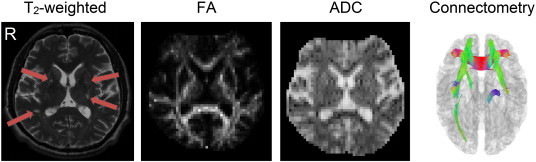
Diffusion MRI connectometry compared with T_2_-weighted images, as well as the ADC and FA maps in patient 2. The T_2_-weighted image shows higher signal intensity at the lesion locations, whereas the ADC map shows a subtle increase and the FA map shows a subtle decrease in the lesion site. None of these three modalities was able to reveal the entirety of the affected tracks. By contrast, connectometry presented the affected tracks that matched the lesion locations.
